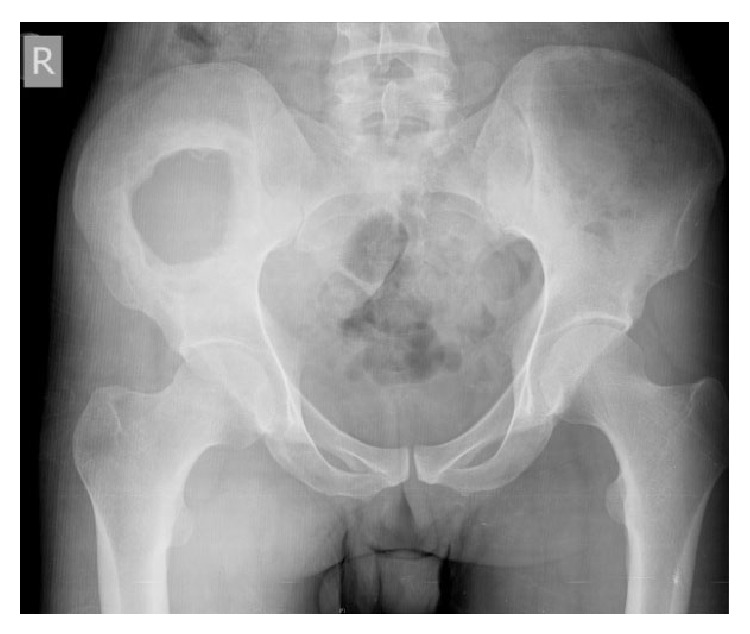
Plain radiograph showing a lytic lesion in the right iliac wing with minimal periosteal reaction.
